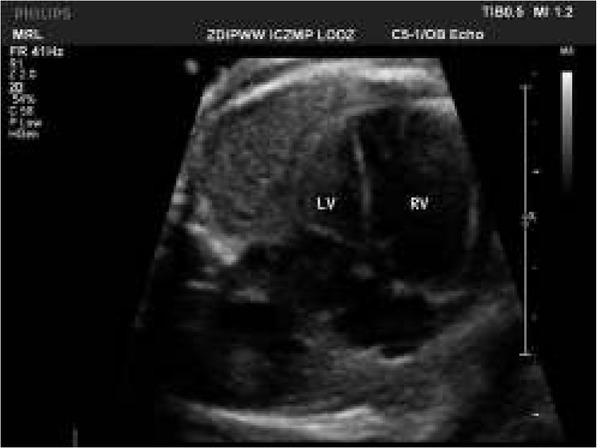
The same patient at 38th weeks of gestation with cardiomegaly, abnormal heart axis, and beginning of fetal congestive heart failure which requires trans-placental therapy for safe pregnancy continuation (fetal echocardiography monitoring and therapy is presented in Table VI). Ryc. 7. Ten sam pacjent w 38 tygodniu ciąży z kardiomegalią, nieprawidłową osią serca, z objawami zaczynającej się niewydolności krążenia wymagającej terapii przezłożyskowej celem bezpiecznego kontynuowania ciąży (opis monitorowania tabela VI).
